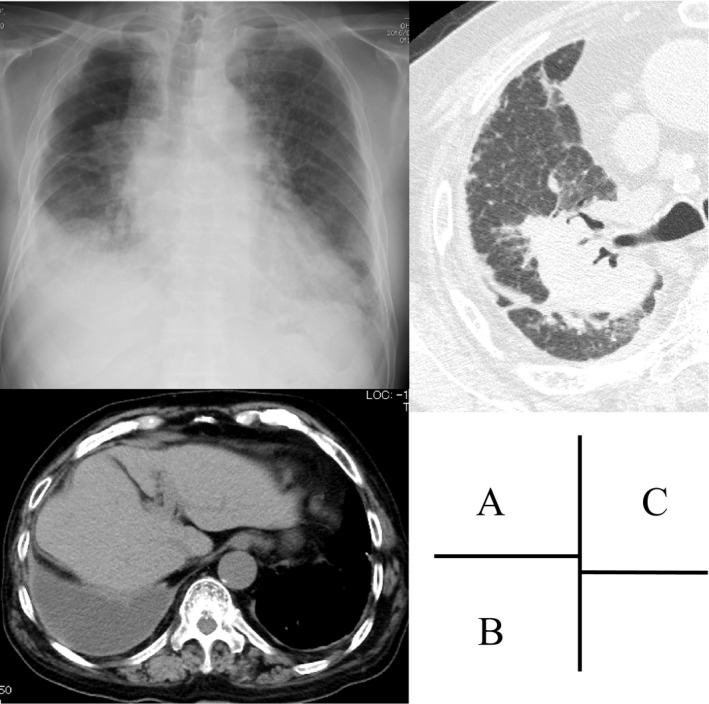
Chest imaging. Chest X‐ray showed a pulmonary nodule and right‐sided pleural effusion (A). Chest computed tomography showed pulmonary nodules, pleural effusion, and thickened pleura (B, C).
